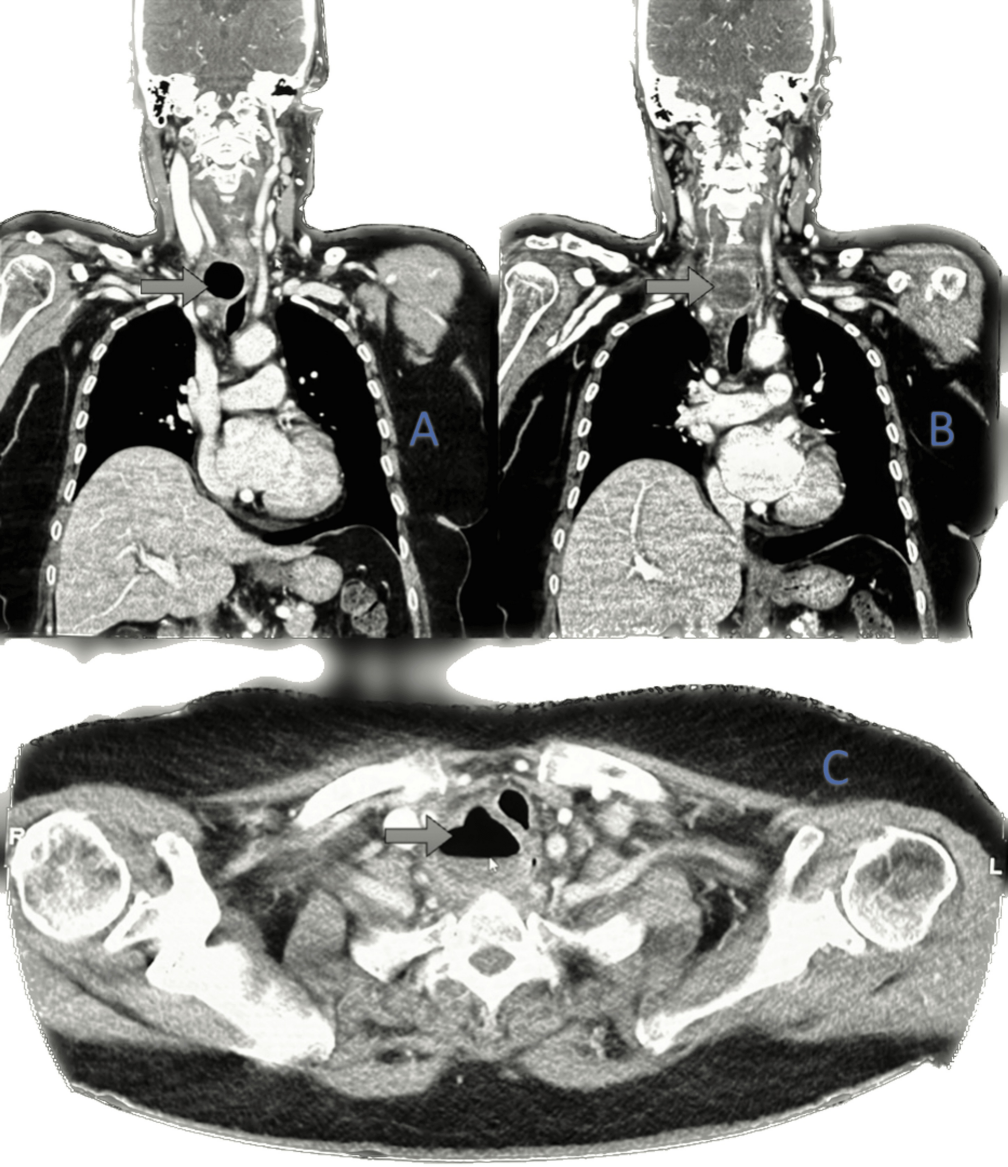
Contrast-enhanced CT scan Showed the right para-esophageal region at the thoracic inlet opposite to the C7-D1 vertebral levels, a well-defined, thick-walled, marginally enhanced collection of air-fluid level. (a) coronal section showing air-filled lesion (b) coronal section showing fluid-filled lesion (c) axial section showing Medially compressing and displacing the esophagus to the contralateral side, also seen as mid-displacing the trachea to the left anterolateral aspect.
